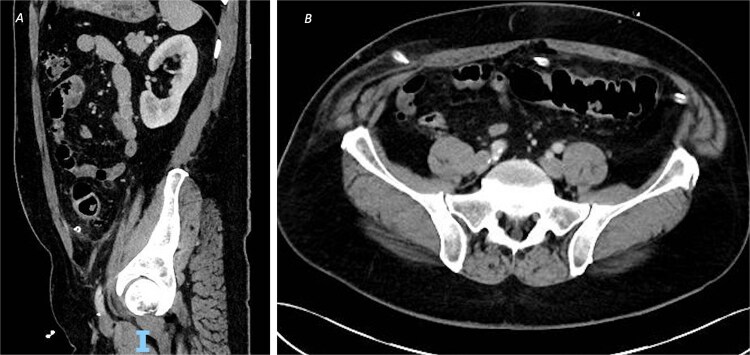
CT of the abdomen and pelvic with contrast on post-operative Day 8 demonstrating resolution of the inflammatory changes with no evidence of leak or abscess formation, from (A) sagittal view and (B) axial view.
